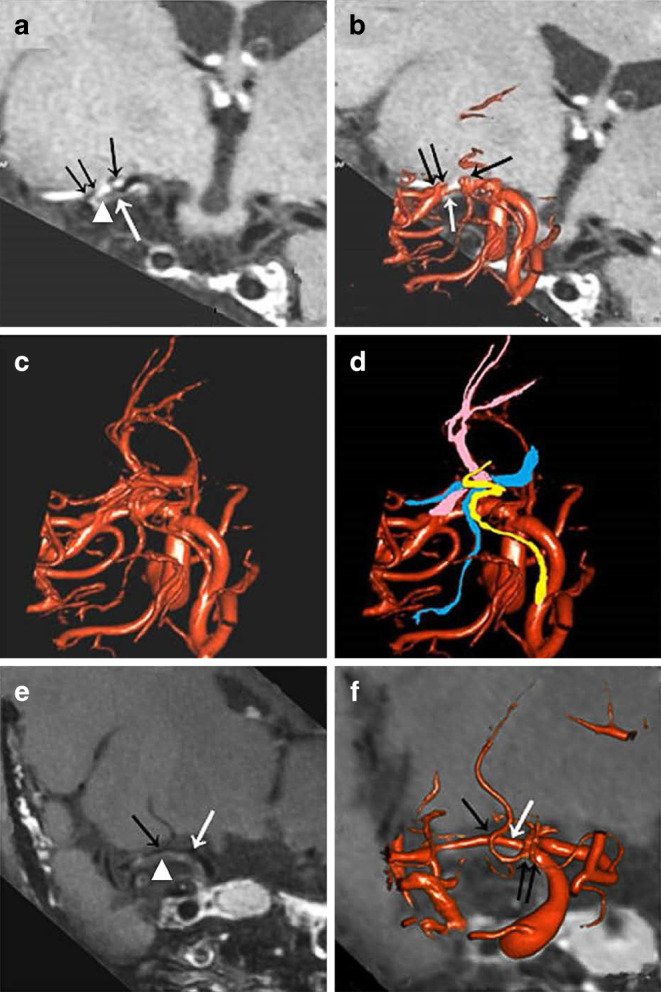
Two cases of misjudgment of the position of a lenticulostriate artery (LSA) orifice with magnetic resonance imaging (MRI). Case 1. a,b The MRI shows the apparent “orifice” of LSA (*black arrow*) that derived from the stenotic middle cerebral artery (MCA) segment, lumen of MCA (*white arrow*) and MCA plaques (*arrowhead*), but misjudges the true orifice of LSA (*double black arrow*). c,d The position of the apparent “orifice” of LSA actually consists of recurrent artery of Heubner (*yellow*) and parallel veins (*blue*) in three-dimensional digital subtraction angiography (3D DSA). The true LSA is located at the distal non-stenotic MCA segment (*pink*). Case 2. e The MRI shows lumen of MCA (*white arrow*), the apparent “orifice” of LSA (*black arrow*) and MCA plaques (*arrowhead*). f The 3D DSA and MRI fusion image confirms that the position of apparent “orifice” of LSA in MRI (*black arrow*) is the intersection of LSA and MCA (*white arrow*). The true orifice of LSA is located at a more proximal section of the MCA (*double black arrow*)
